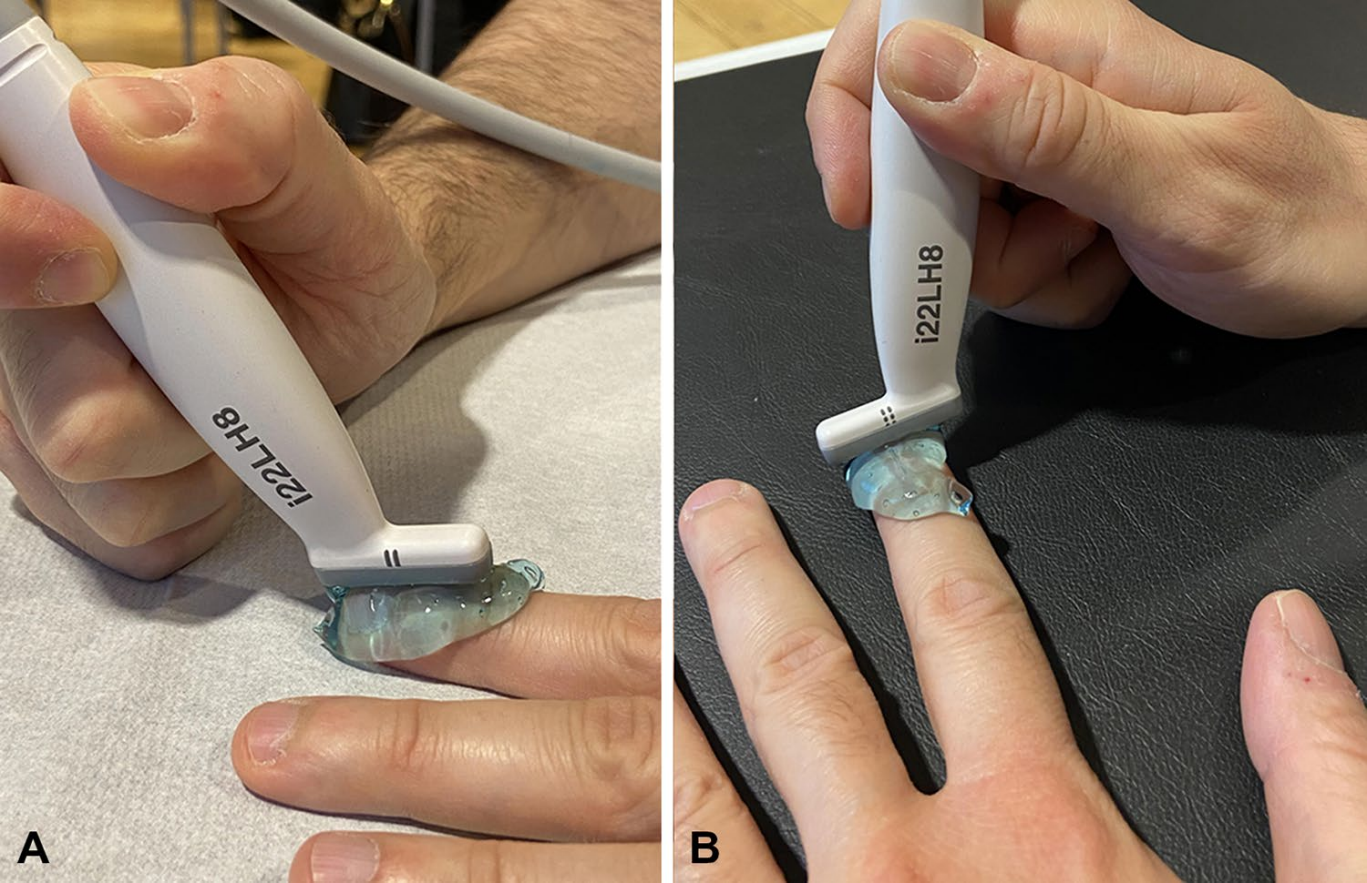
Position of the probe for longitudinal and transverse view. In A the probe is gently positioned in the longitudinal middle axis of the finger. A consistent amount of gel is place above the nail and then the probe is placed, avoiding compression. As showed, there is no direct contact between the probe and the nail plate, avoiding compression. Placing ultrasound gel is crucial and an adequate amount should exceed over the probe edges, as showed in A and B. In B, the probe is placed at the middle portion of the nail, to an angle of 90° of longitudinal middle axis. Also in this case the gel placement allow a full cover of the nail reducing artifacts and potential errors in the measurements
